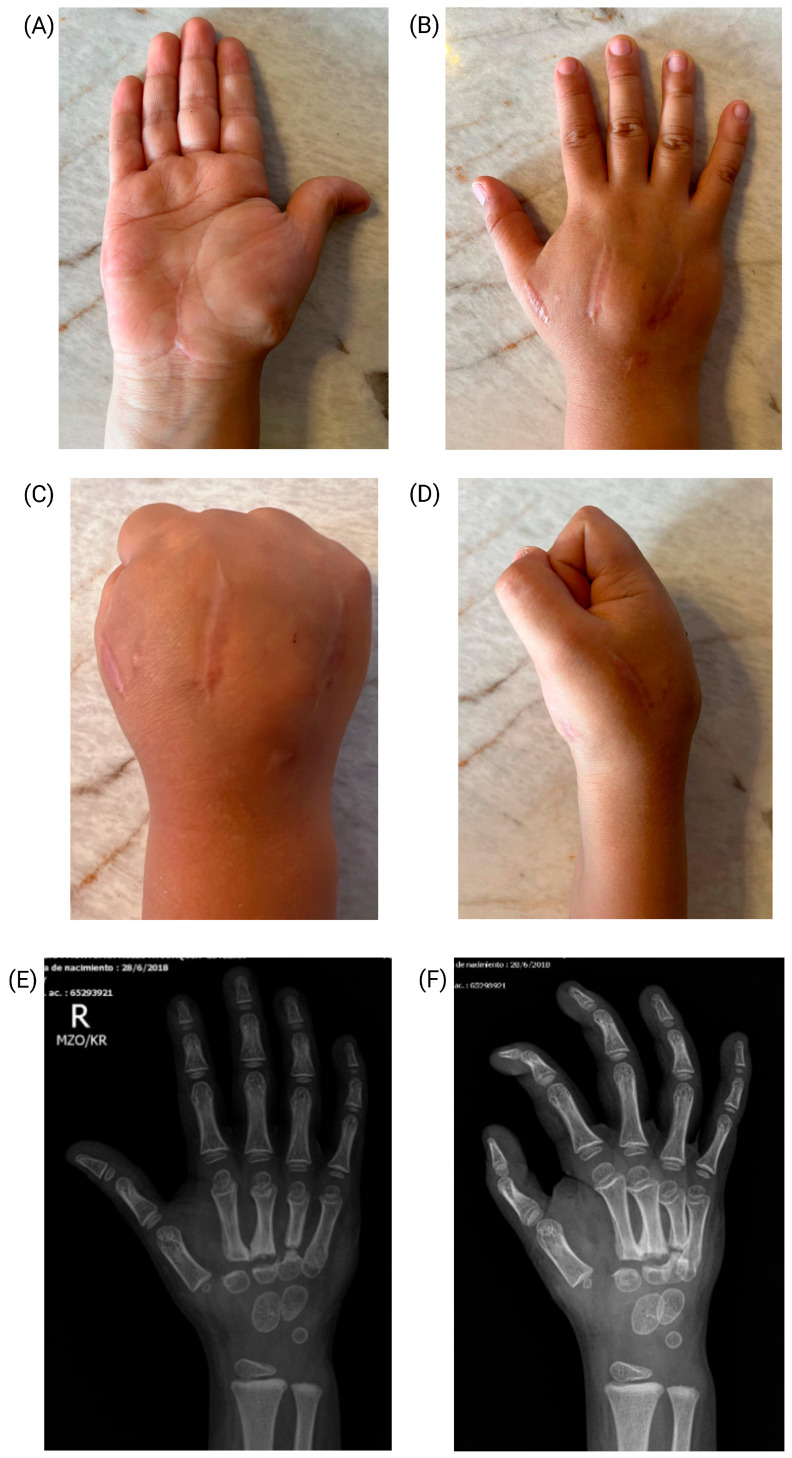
Functional recovery of the hand. (A) Palmar extension of the hand. (B) Dorsal extension of the hand. (C) Hand flexion in dorsal view. (D) Hand flexion in lateral view. (E) Anteroposterior radiograph of the hand showing callus formation and bone remodeling. (F) Oblique radiograph of the hand showing callus formation and bone remodeling.
